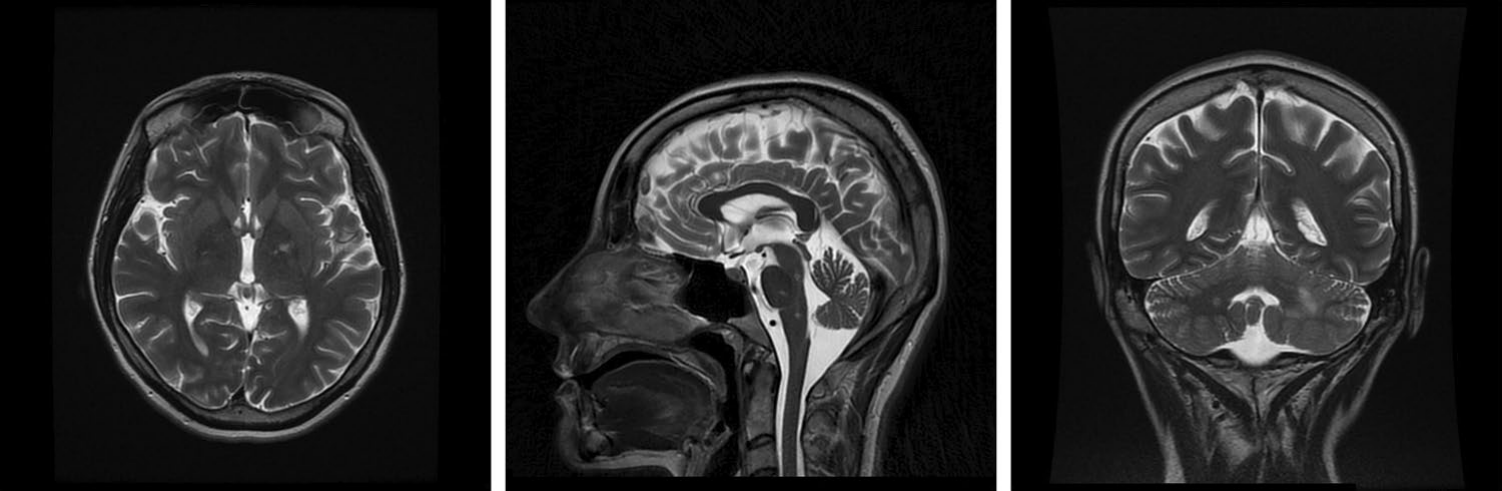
Patient B: T2-weighted brain MRI, transverse, sagittal, and coronal planes
